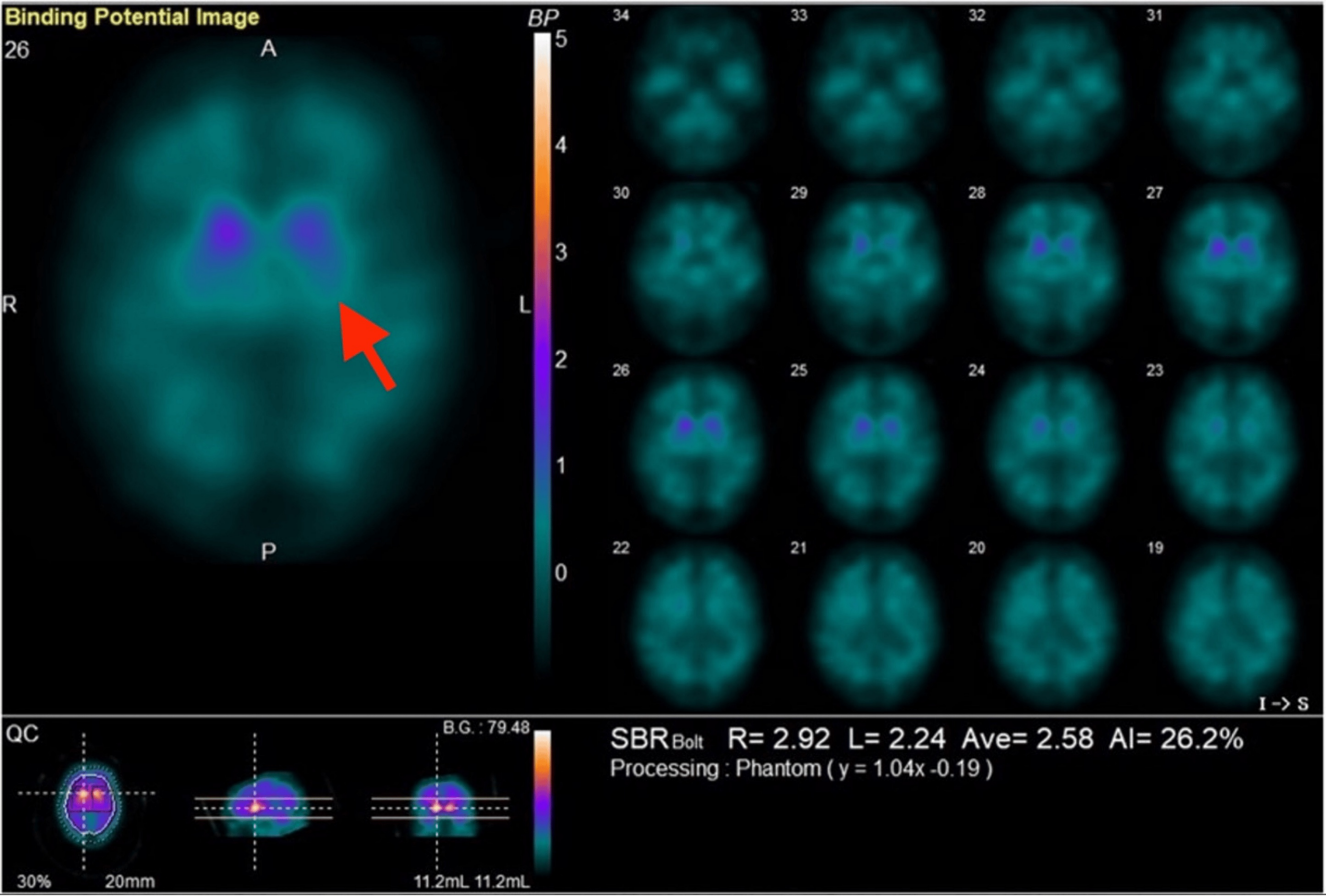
Dopamine transporter SPECT (DaTscan) The scan demonstrates reduced specific binding ratios (SBR) in the striatum, particularly in the left basal ganglia (red arrows), consistent with the patient's right-sided motor symptoms. SPECT: Single-photon emission computed tomography.
